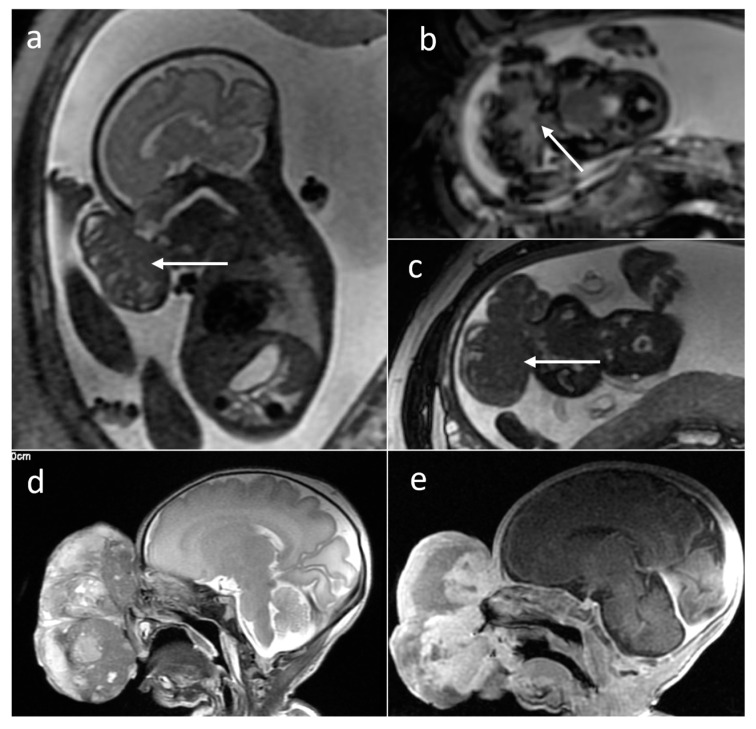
Extra-pleural hemangiopericytoma/solitary fibrous tumor in a fetus (arrows). A mass of mixed signal intensity deriving from the face, with peripheral deposits of hemosiderin. (a) Sagittal SSFSE/T2WI; (b) axial GRE EPI; (c) axial FIESTA. Postnatal MRI: (d) sagittal T2WI; (e) CE-T1WI+Gd showing inhomogeneous contrast enhancement.
